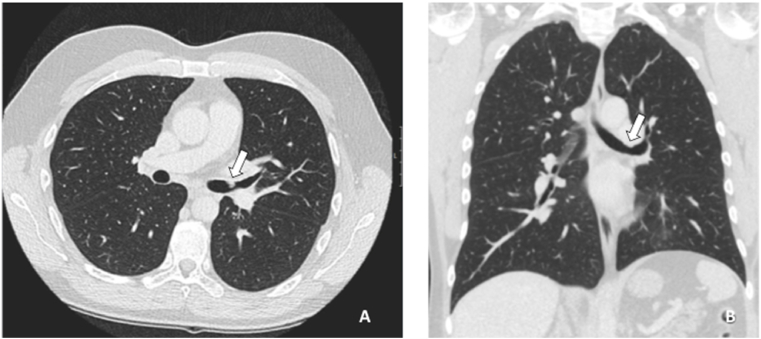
Computed Tomography A; transversal and B; coronal images of the chest after initial bronchoscopy showing a remaining lesion in the left main bronchus (arrow).
